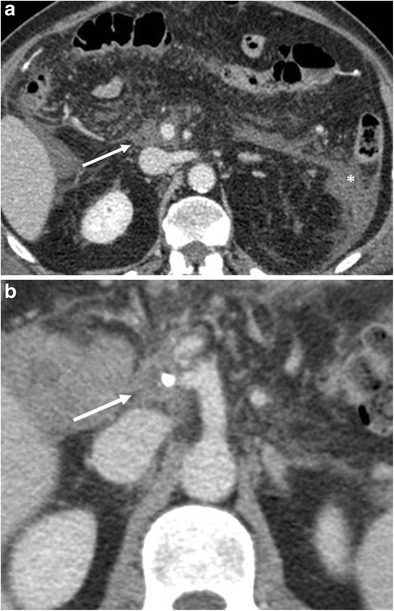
Perivascular cuffing. a, b) Axial CT images show a thickening (white arrows in a and b) of the fat tissue surrounding the superior mesenteric vessels in a and caeliac trunk in b. This stranding can be very focal and mass-like. In a, a postoperative reactive thickening of the anterior left pararenal fascia and a fluid collection on the same side (*) are visible
